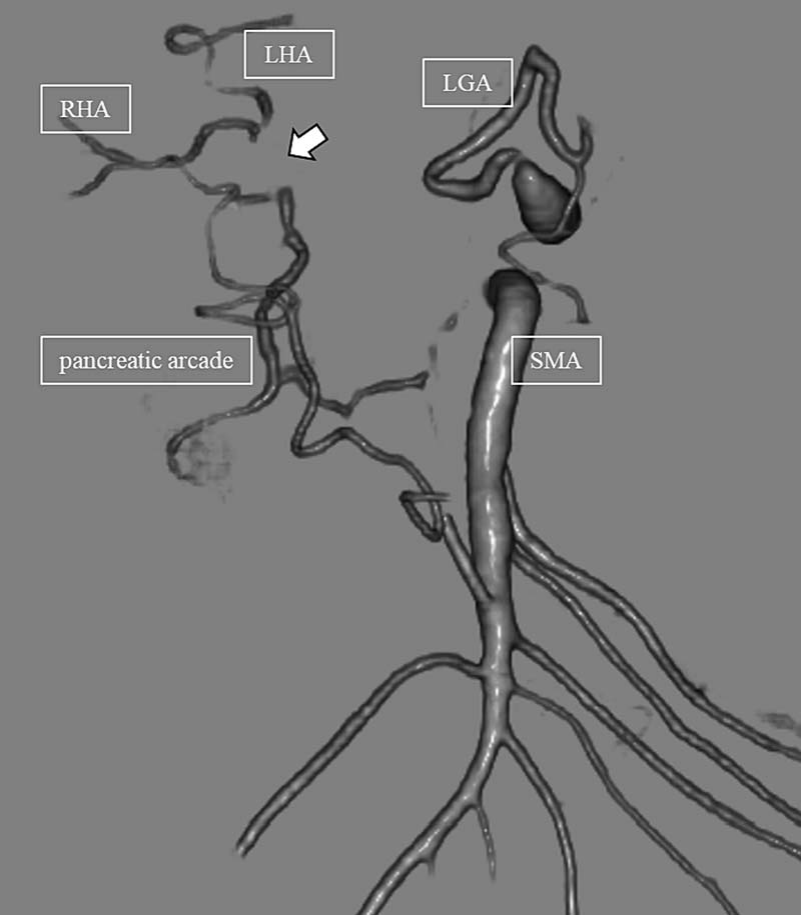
CT on postoperative Day 61. Although blood flow in the PHA could not be confirmed (arrow), the right and left hepatic arteries are preserved.
